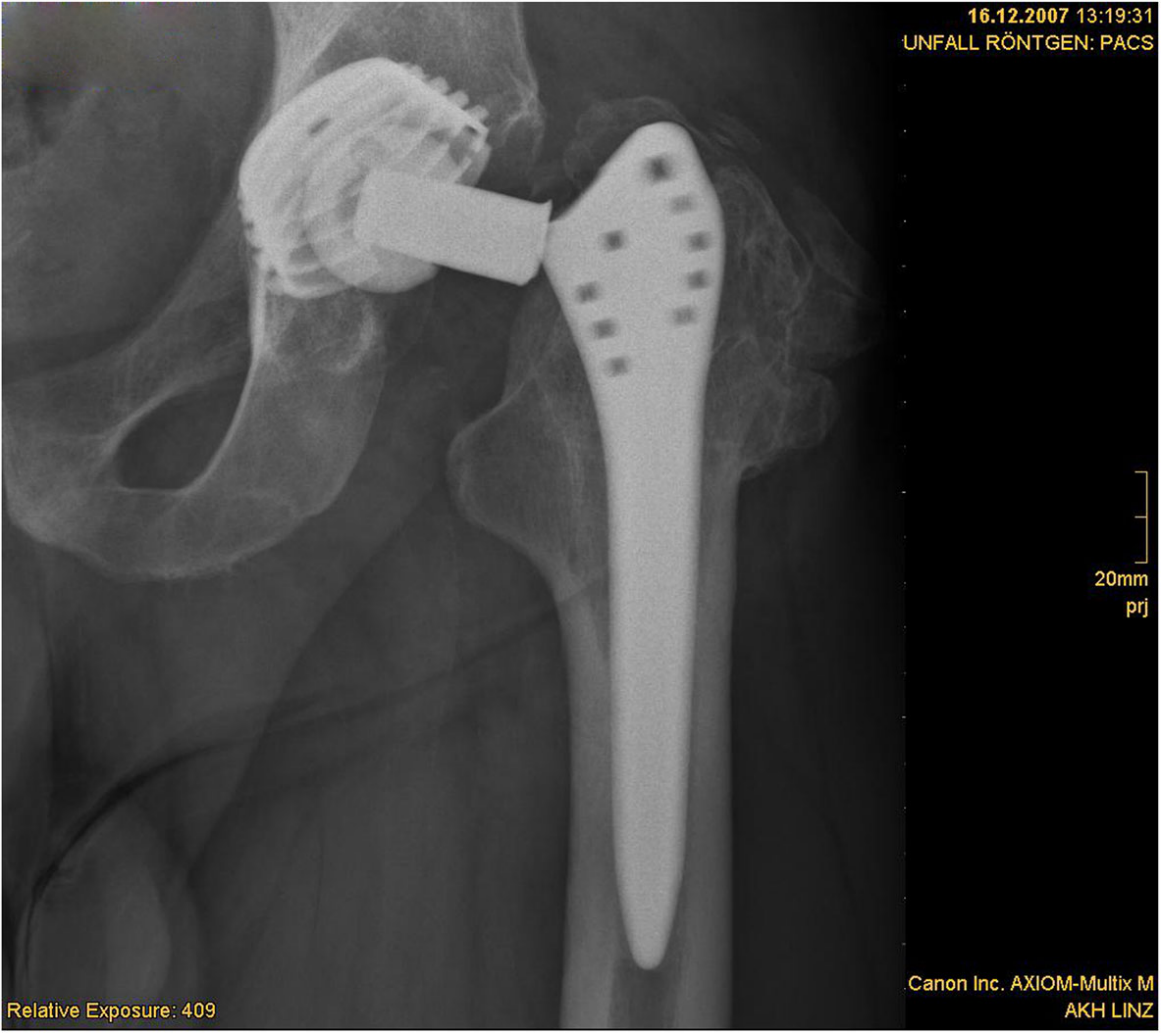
Radiograph showing implant fracture after 20 years
